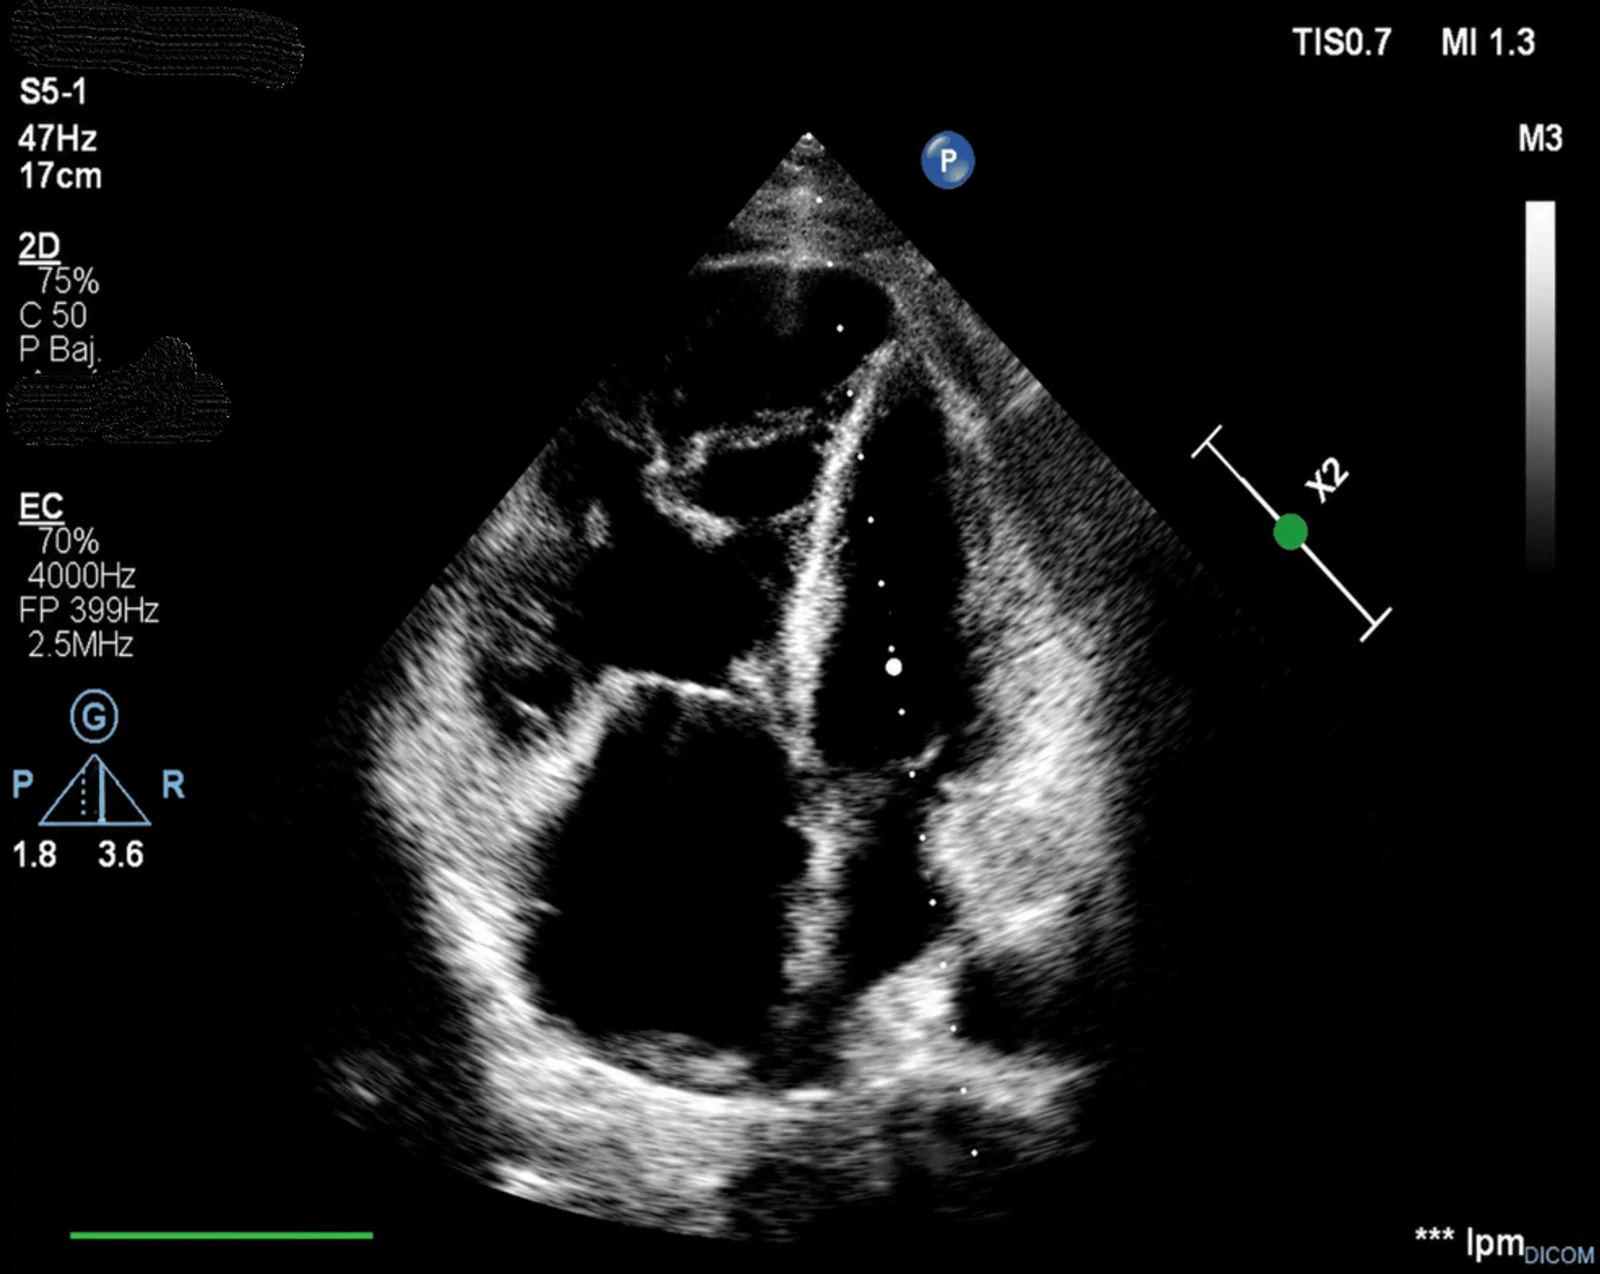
Transthoracic echocardiography demonstrating a severely dilated right ventricle (basal diameter, 54 mm; mid-cavity diameter, 41 mm. Quantitative right ventricular assessment showed a fractional area change (FAC) of 45%, free-wall longitudinal strain of -28%, tricuspid annular plane systolic excursion (TAPSE) of 17 mm, tissue Doppler S′ velocity of 12 cm/s, and an estimated pulmonary artery systolic pressure (PASP) of 42 mmHg.
